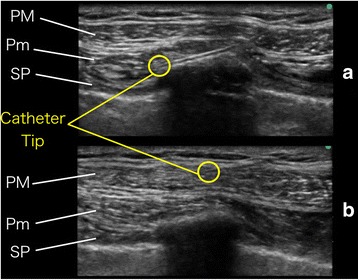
Ultrasound images related to PECS block. a An image just after the catheterization was obtained with catheter tip being located between the pectoralis minor and the serratus muscles on forth rib. b A postoperative image was obtained with displacement of the catheter tip deep in the pectoralis major muscles. PM pectoralis major muscle, Pm pectoralis minor muscle, SP serratus muscle
